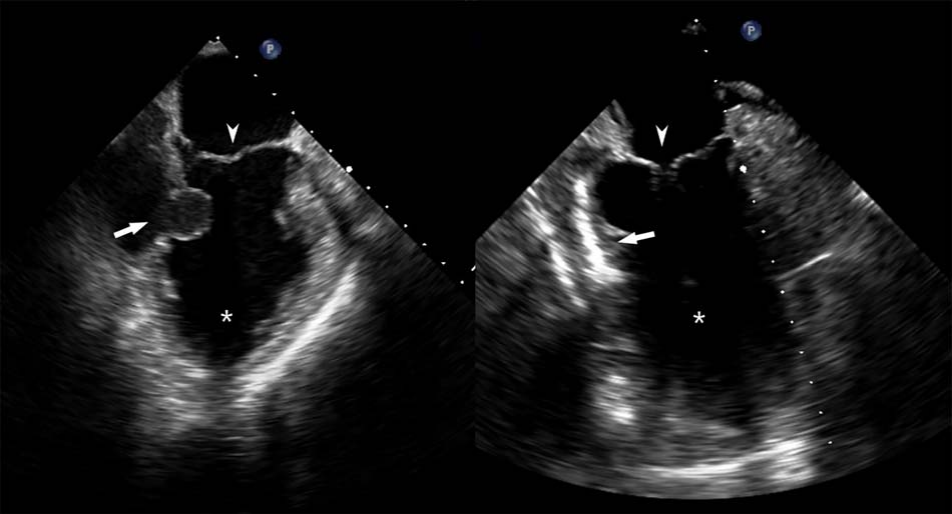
Pre- and post-repair transesophageal echocardiography images; leftward image: asterisk = left ventricular cavity, arrow = ventricular wall defect, arrowhead = mitral valve; rightward image: asterisk = left ventricular cavity, arrow = ventricular wall patch closure, arrowhead = mitral valve.
